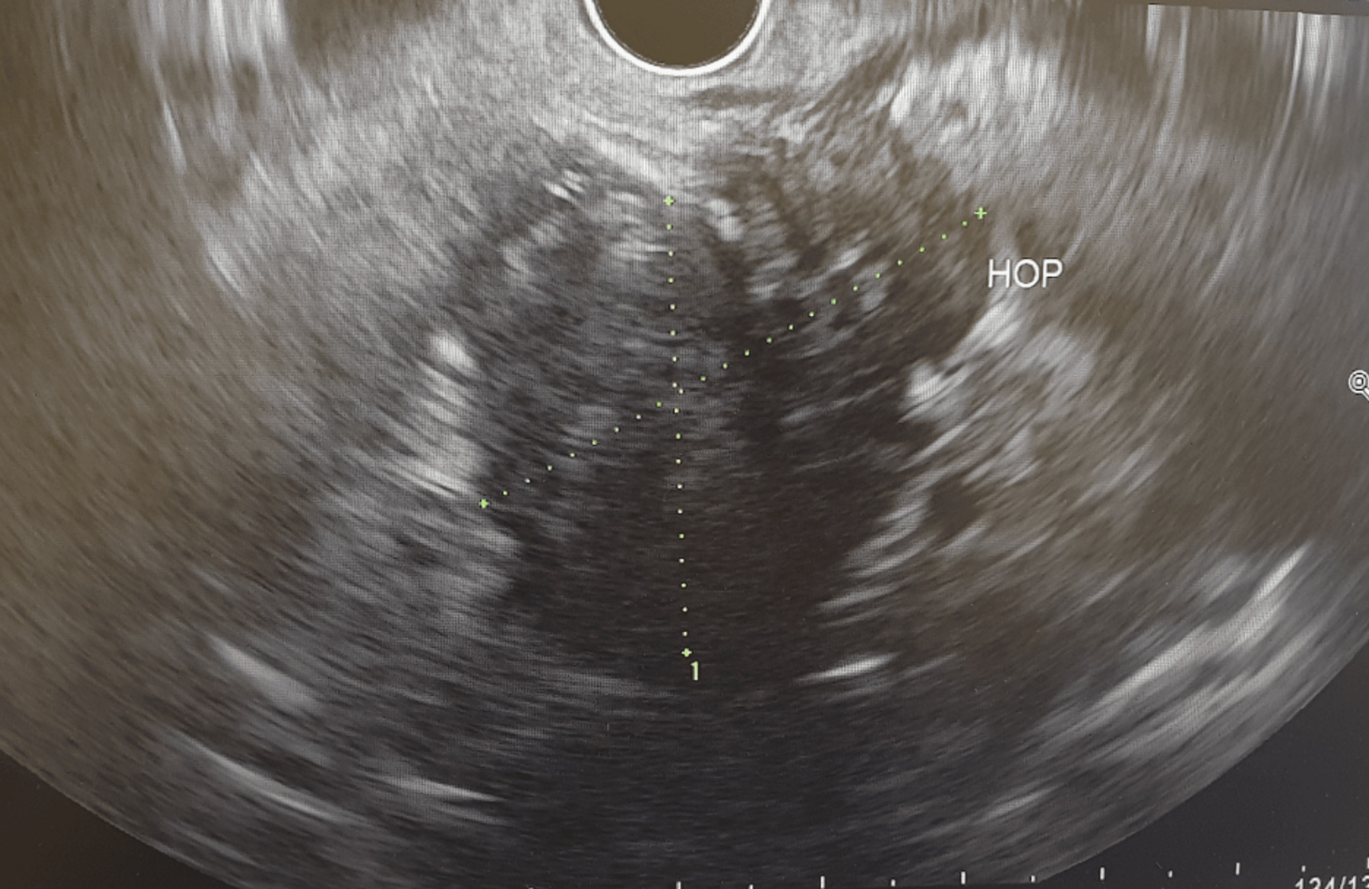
Endoscopic ultrasound showing pancreatic head mass.
